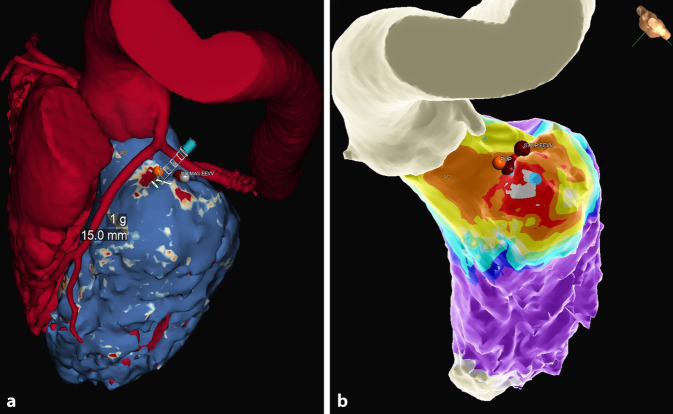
A 14-year-old boy with ventricular tachycardia originating from the left ventricular summit. a Cardiac computed tomography (CT) scan of the patient to identify coronary arteries and analyzed with ADAS 3D software to identify regions of possible fibrosis. b Ensite X local activation time map integrated with the ADAS 3D CT scan. Note how the earliest activation region corresponds to regions of possible fibrosis identified with ADAS 3D. Successful ablation points shown on the map
